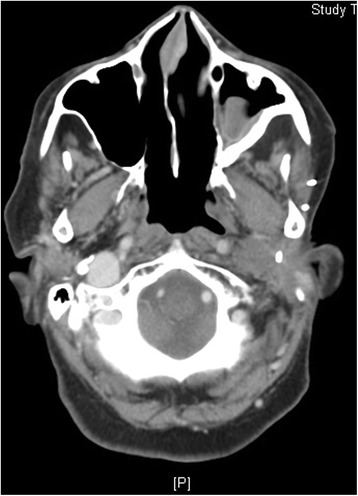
Magnetic resonance imaging (MRI) face showing recurrent mass in parotid bed
